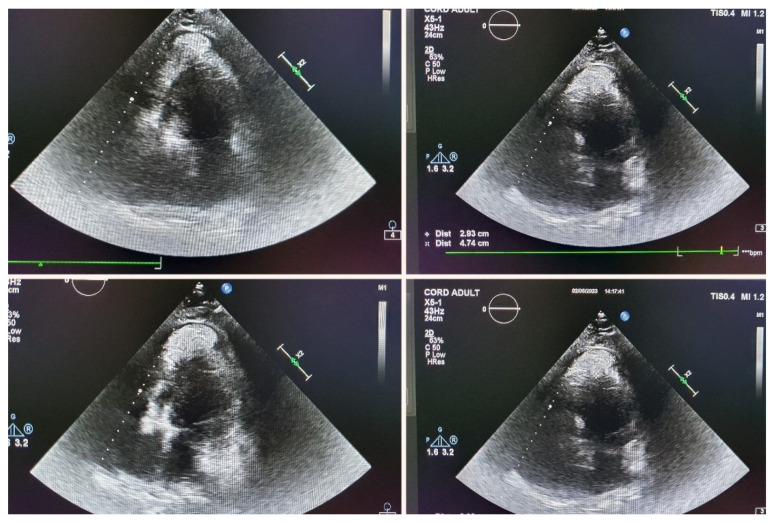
Echocardiographic evaluation.
